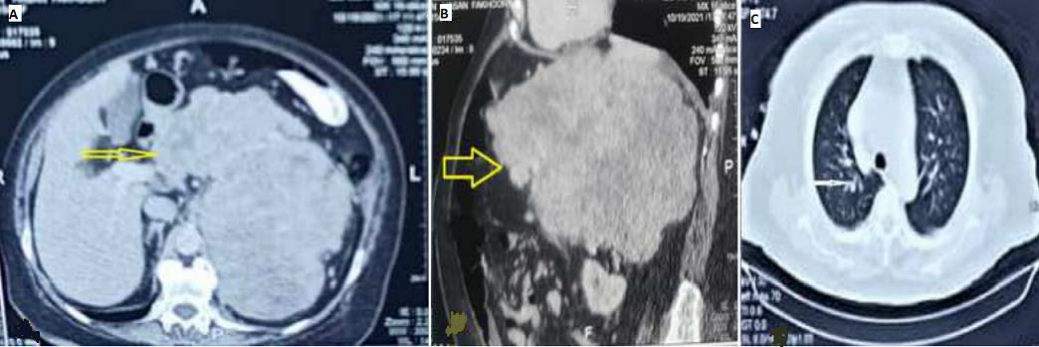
A) axial; B) sagittal views of abdominal CT scan revealing a large mixed echogenic mass arising from the gastric wall involving the surrounding organs (arrow); C) showing lung metastasis (arrow)
